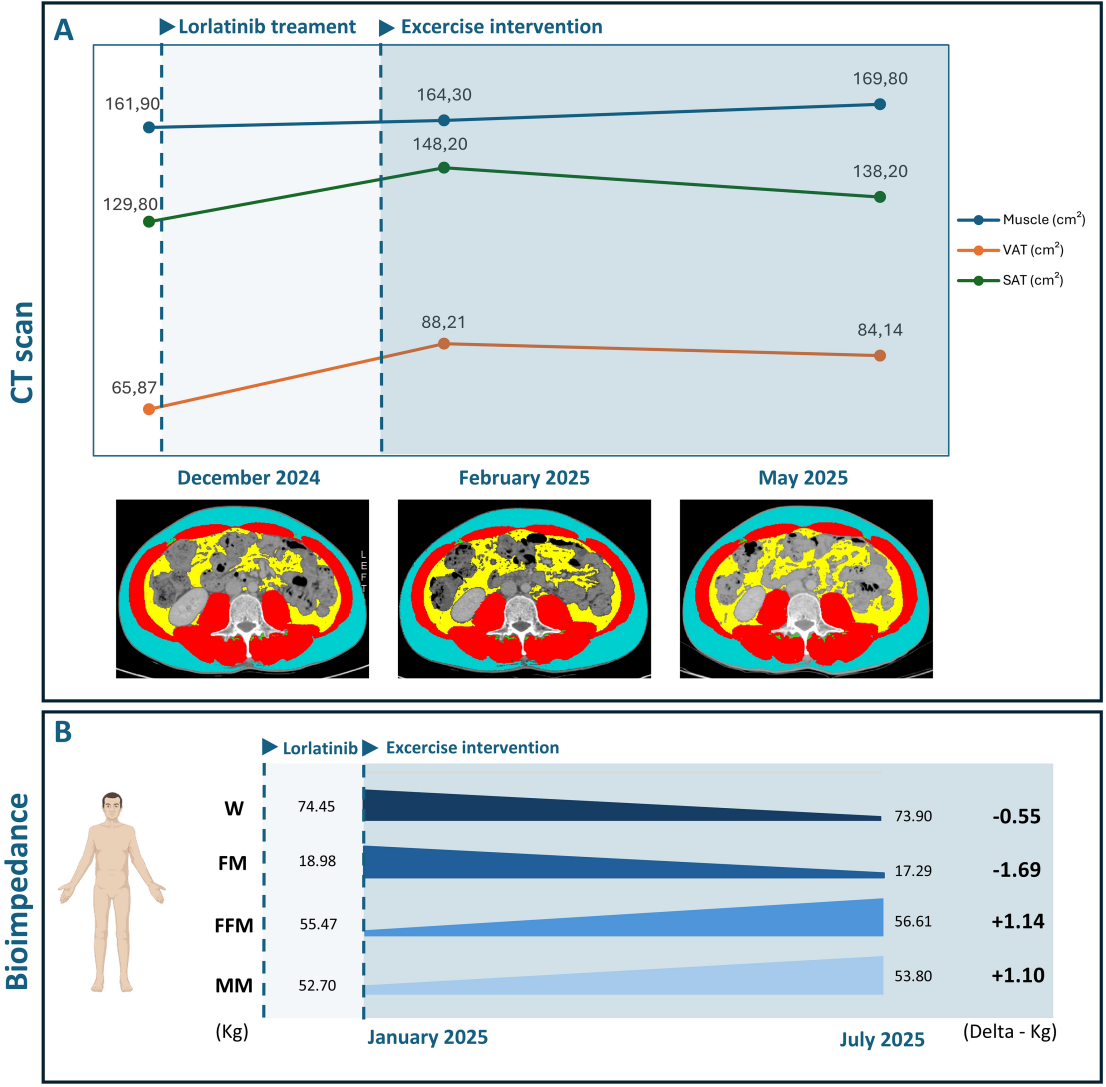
(A) Body composition changes since diagnosis and during exercise intervention, assessed via CT scans. (B) Body composition changes during exercise intervention, assessed via bioimpedance analysis.
